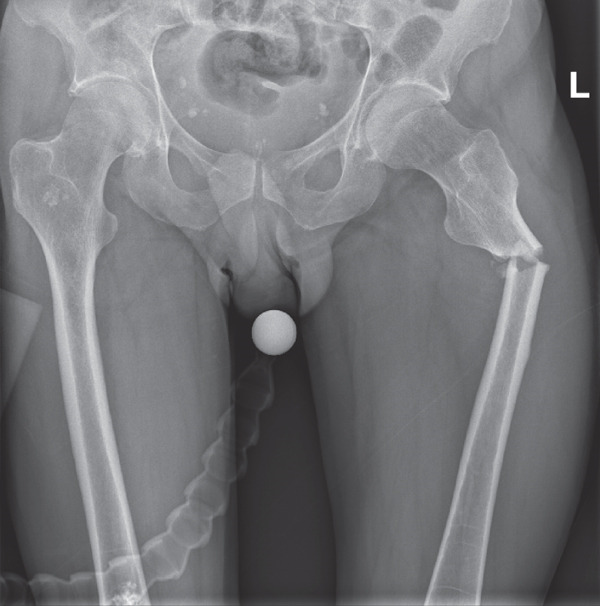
Initial anteroposterior radiograph following presentation to the emergency department showing a complete lateral cortex transverse fracture of the left femur.
